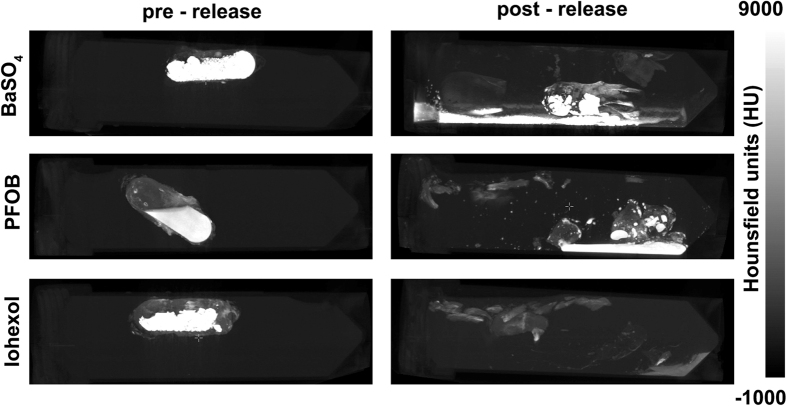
CT images show iron-oxide wax coated capsules containing CT contrast agents before and after triggered release (60 minutes). Post-release images show the distribution of the released contrast agents.
